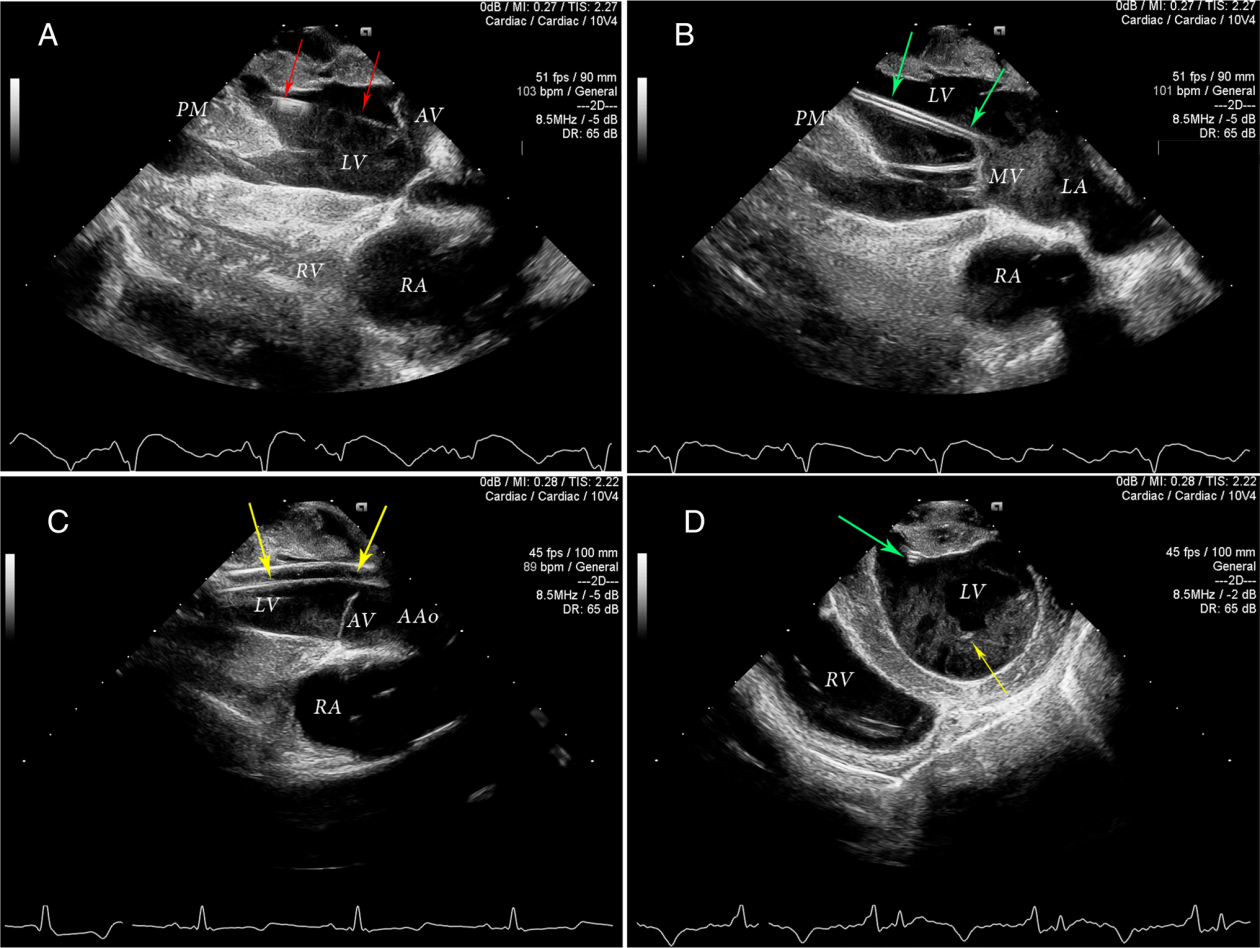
Sequential two-dimensional greyscale epicardial echocardiographic image acquisition in correctly placed elements of Impella CP implantation kit. LV – left ventricle, RV – right ventricle, LA – left atrium, RA – right atrium, AV – aortic valve, MV – mitral valve, Aao – ascending aorta, PM – papillary muscle. A Adequate position of the 0.035-inch guidewire (red arrows) across the aortic valve and along LV long axis between PMs, with the tip in LV apex. B Adequate position of the diagnostic catheter (green arrows) with longitudinal image projecting from the level of the anterior mitral leaflet towards LV apex. C Correct position of the Impella CP (yellow arrows) across the aortic valve and along LV axis. The base and mid- LV cavity is well visualized. D Short-axis confirmation view of the Impella CP catheter position (green arrow) adjacent to the base of the anterolateral PM, and the 0.018-inch guidewire (yellow arrow) in the mid-left ventricle
